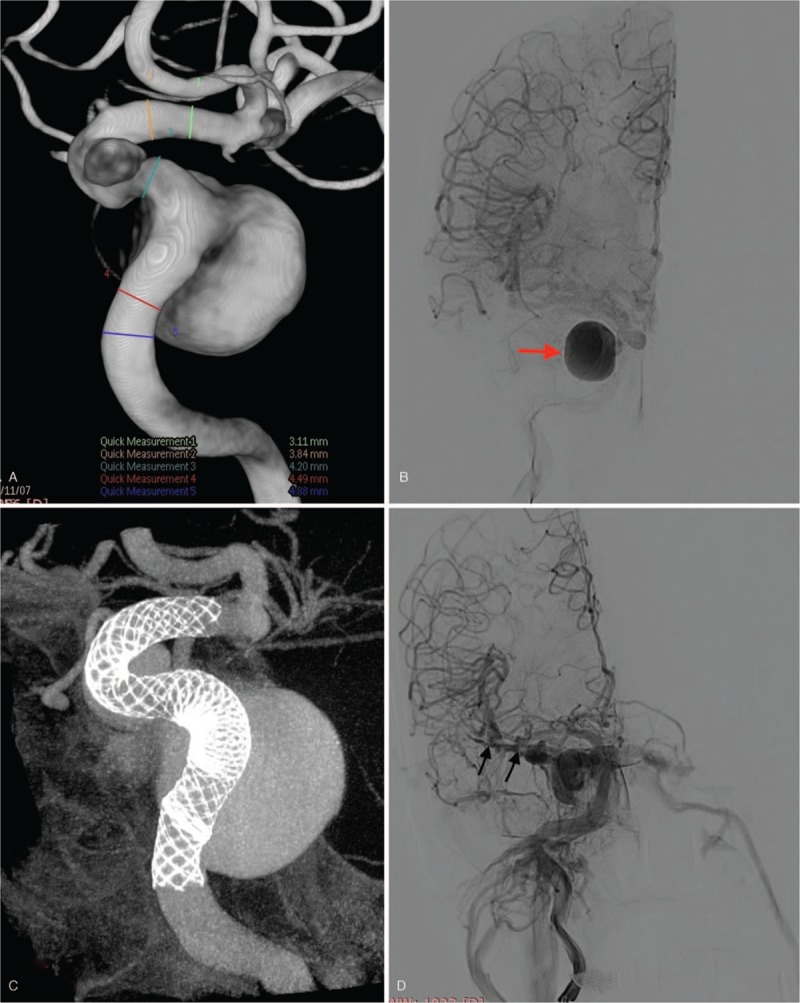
A: 3D reconstruction disclosed a small clinoidal segment aneurysm and a large cavernous segment aneurysm of the right internal carotid artery (ICA). B: frontal view: post-operative angiogram showed obvious contrast retention in the large aneurysm sac (red arrow), while not in the small. C: The Vaso-CT displayed satisfactory apposition of the stent which bridged the two aneurysmal necks completely. D: Frontal view: sphenoparietal sinus (SS) or cortical vein drainage (black arrow) of the CCF following PLED. E: Lateral view: superior ophthalmic vein (SOV, white arrow), pterygoid plexus (PP, white asterisk), inferior petrosal sinuses (IPS, black arrow) and superior petrosal sinuses (SPS, red arrow). F: The microcatheter tip locates in the origin of right SOV (white arrow). G: Frontal view: Showing a little SS (white arrow) and PP drainage (white asterisk) after the second procedure. H: Lateral view: Showing a little SS (white arrow) and PP drainage (white asterisk) after the second procedure. I: Frontal view of DSA during 4-month follow-up showing complete occlusion of the CCF. J: Lateral view of DSA during 4-month follow-up showing complete occlusion of the CCF.
